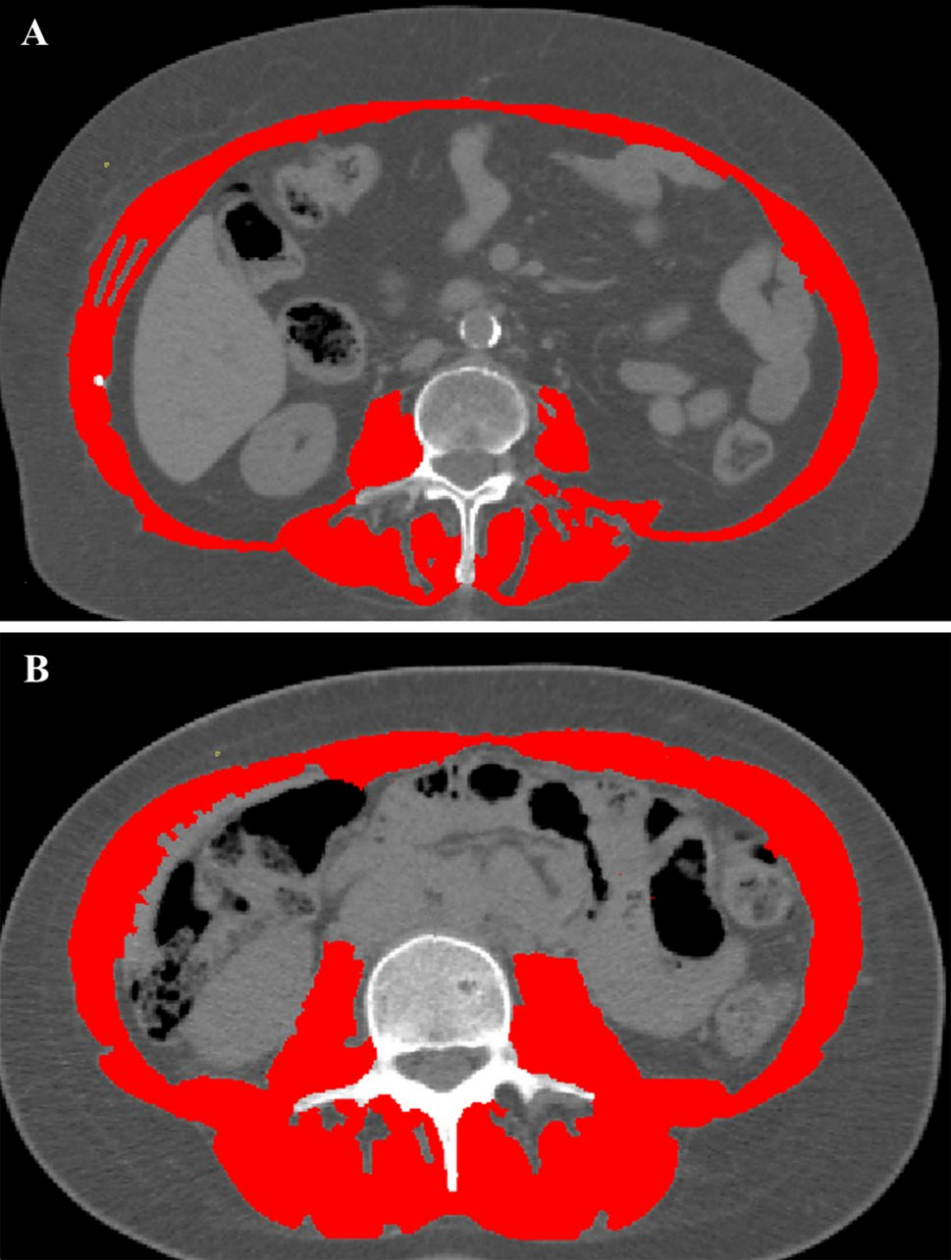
Computed tomography images of typical sarcopenic (image A) and non-sarcopenic (image B) patient. Skeletal muscle area (which is shown in red) is reduced in patient A, and this is especially visible in the paraspinal muscles which are larger and less infiltrated by intramuscular fat. A skeletal muscle index of 38 cm^2^/m^2^ and of 58 cm^2^/m^2^ were calculated using the CSA and height (CSA/height^2^) of patients A and B, respectively
